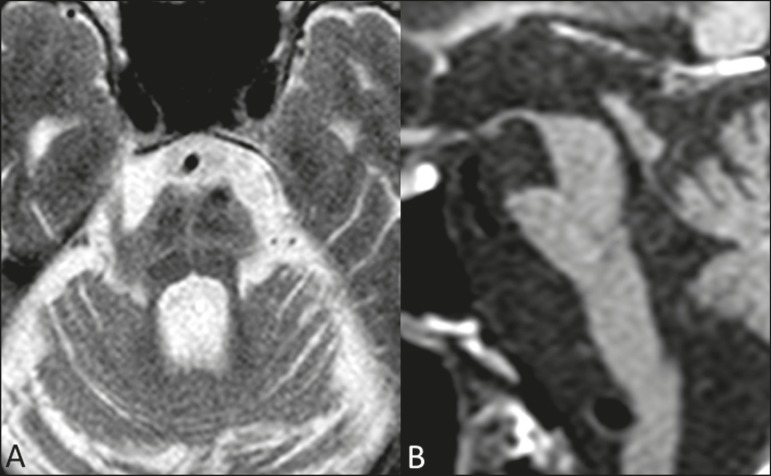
Morphological and signal changes on a conventional MRI scan of a
